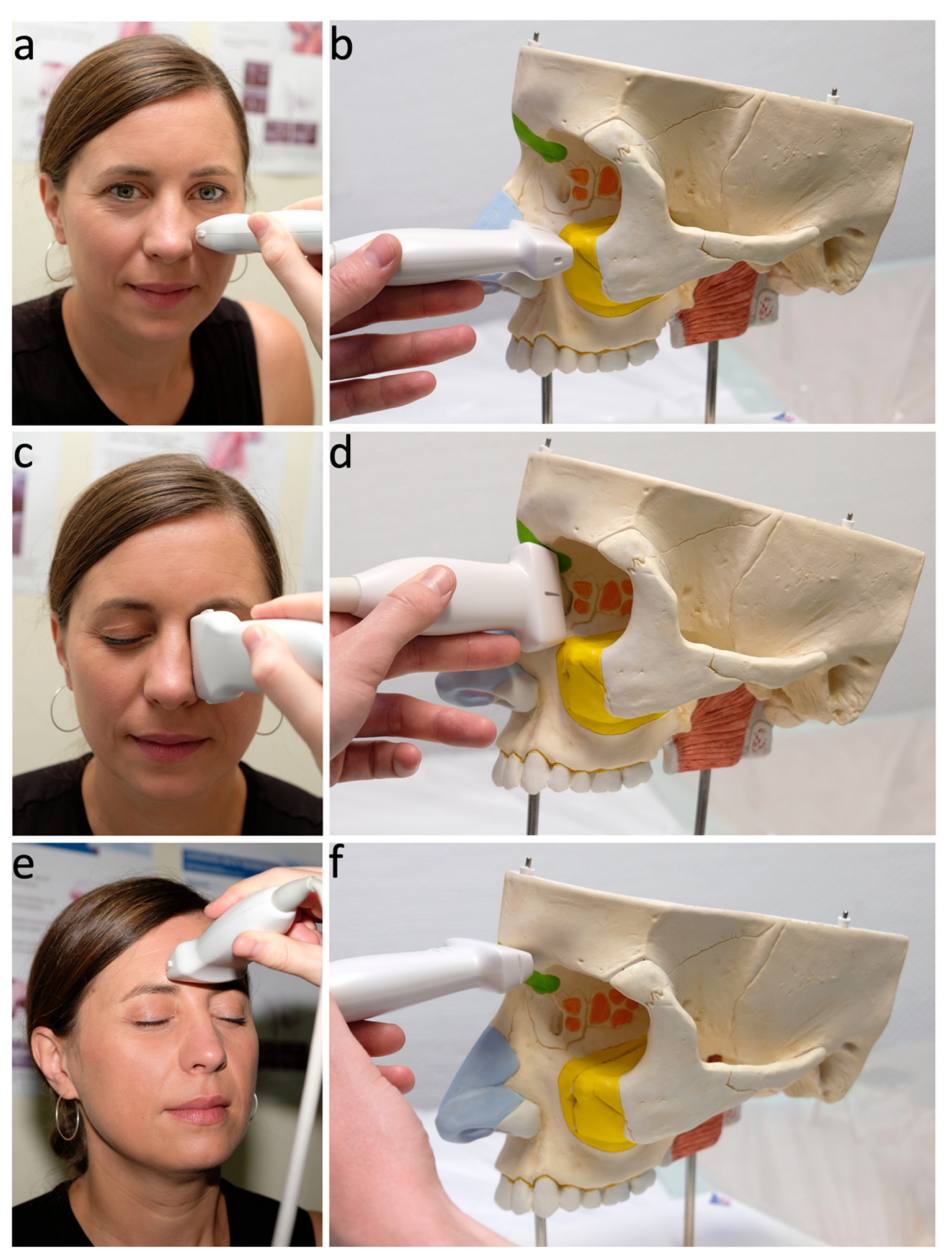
Transducer-positions in B-mode ultrasound of the paranasal sinuses. (a,b): Examination of the maxillary sinus in the axial plane. The transducer is positioned horizontally below the orbit on the anterior wall of the maxillary sinus. (c,d): Examination of the ethmoid sinus in a parasagittal plane. The transducer is positioned vertically in the medial corner of the eye following the course of the medial orbital wall. (e,f): Examination of the frontal sinus in the axial plane. The transducer is positioned horizontally in the lower midline of the forehead.
